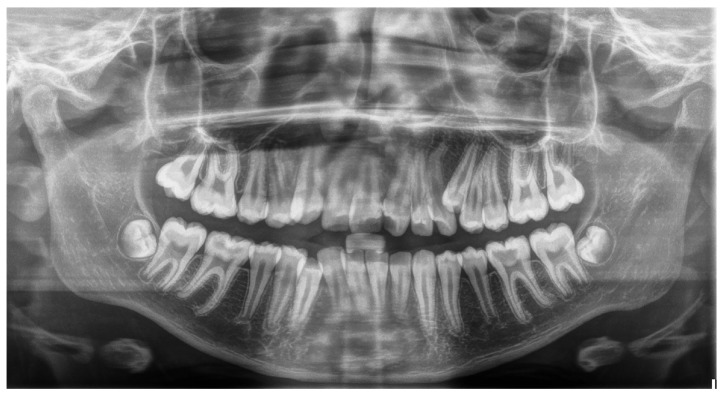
Panoramic radiograph of a patient with supernumerary maxillary left premolar.
